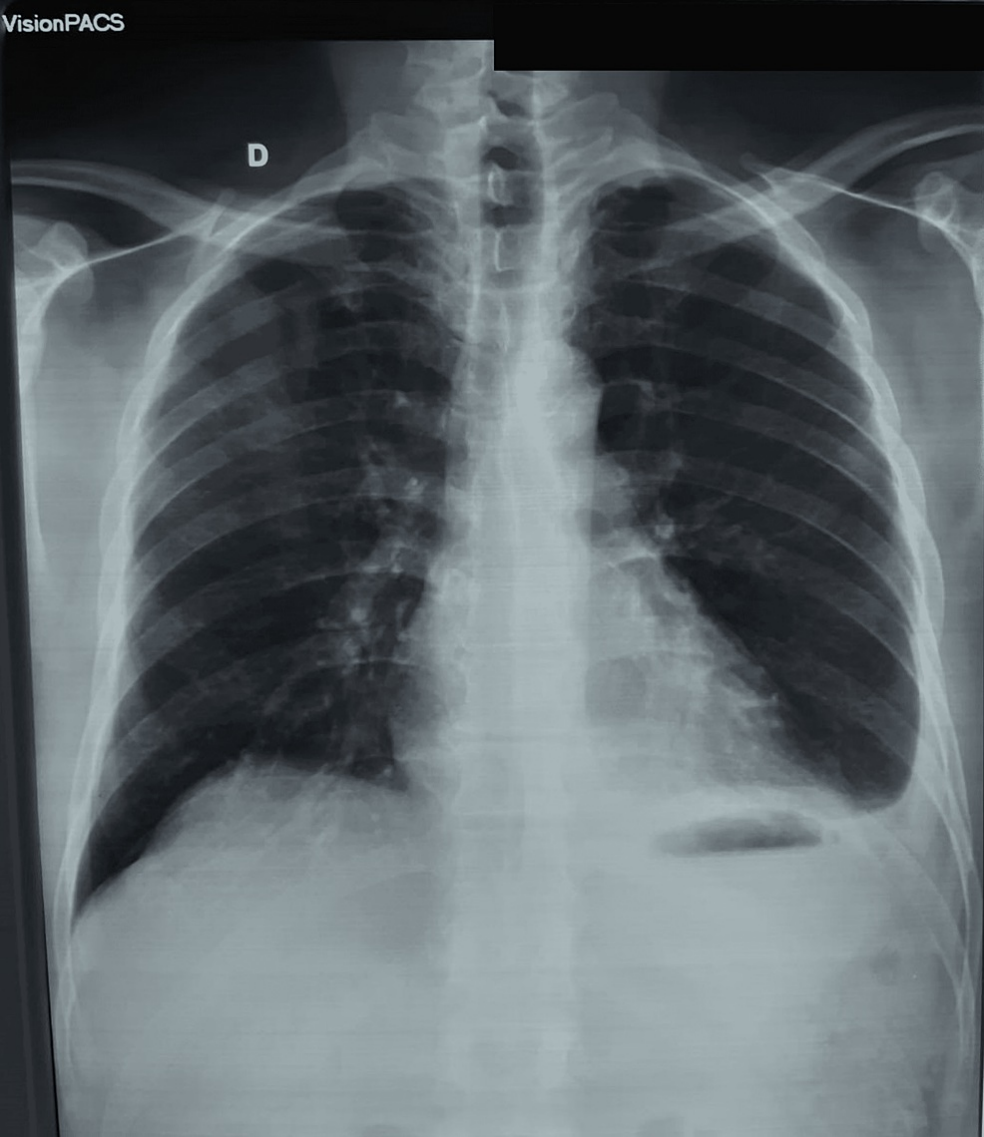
Chest x-ray showing mild unilateral left pleural effusion
